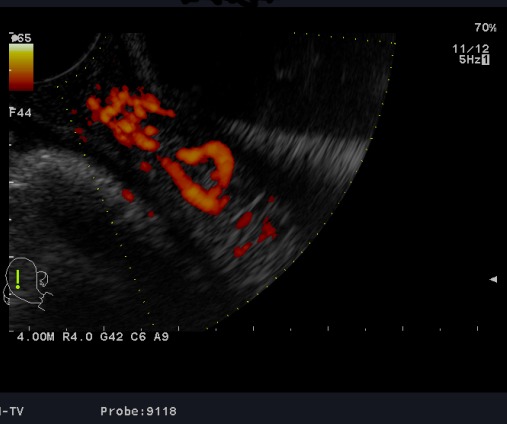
Uterine artery pseudoaneurysm; examination with color Doppler transvaginal US shows spining blood flow
